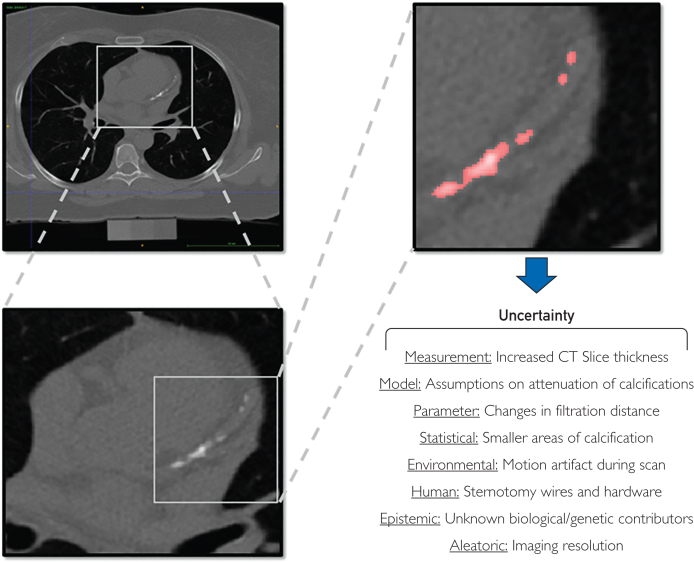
Noncontrast computed tomography (CT) coronary artery calcium scan. How can topological data analysis assess coronary artery calcium? What types of uncertainty should be considered when evaluating the model output?
